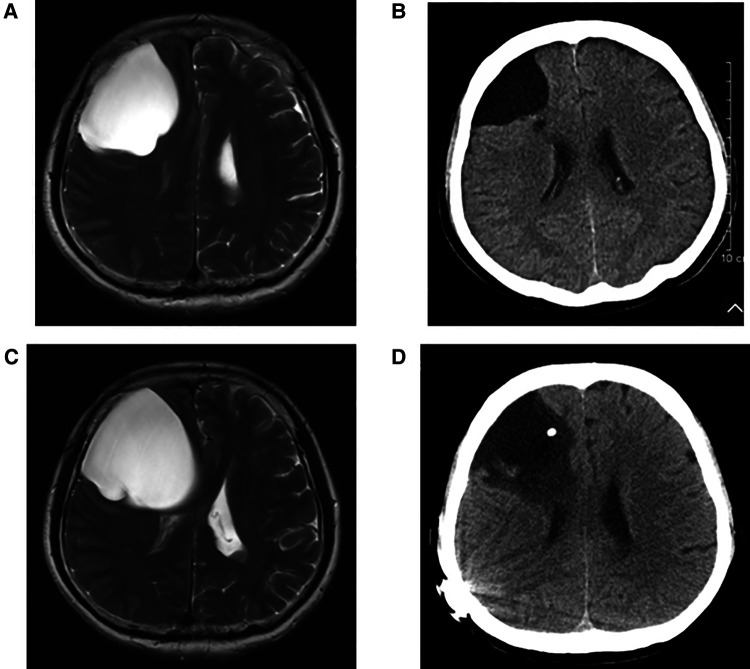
(A) Preoperative T2 MRI image of No. 4 patient. (B) CT image of No. 4 patient at 1 year and 4 months after neuroendoscopic surgery. (C) T2 MRI image of No. 4 patient at 2 years and 6 months after neuroendoscopic surgery. (D) CT image of No. 4 patient after intracranial arachnoid cyst-peritoneal shunt.
